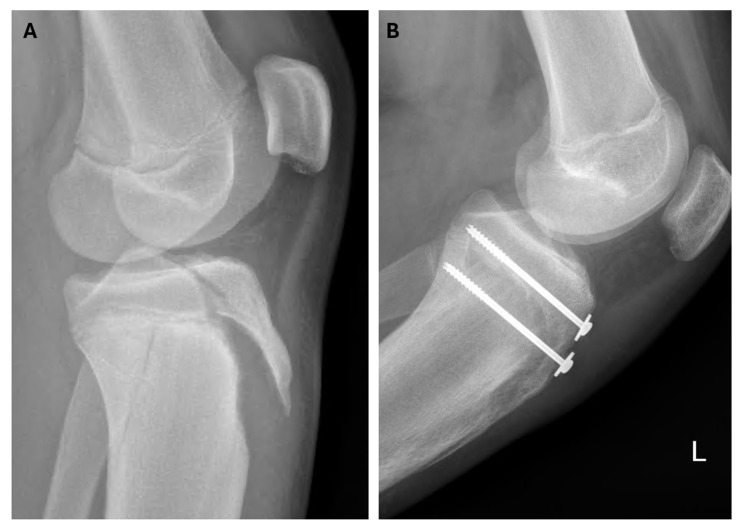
Radiographic images of a type V tibial tuberosity fracture. (A) Preoperative radiograph of a type V tibial tuberosity fracture with extension of the fracture line into the tibial metaphysis. (B) Postoperative radiograph demonstrating a healed fracture 7 weeks after surgery. L indicates left side.
